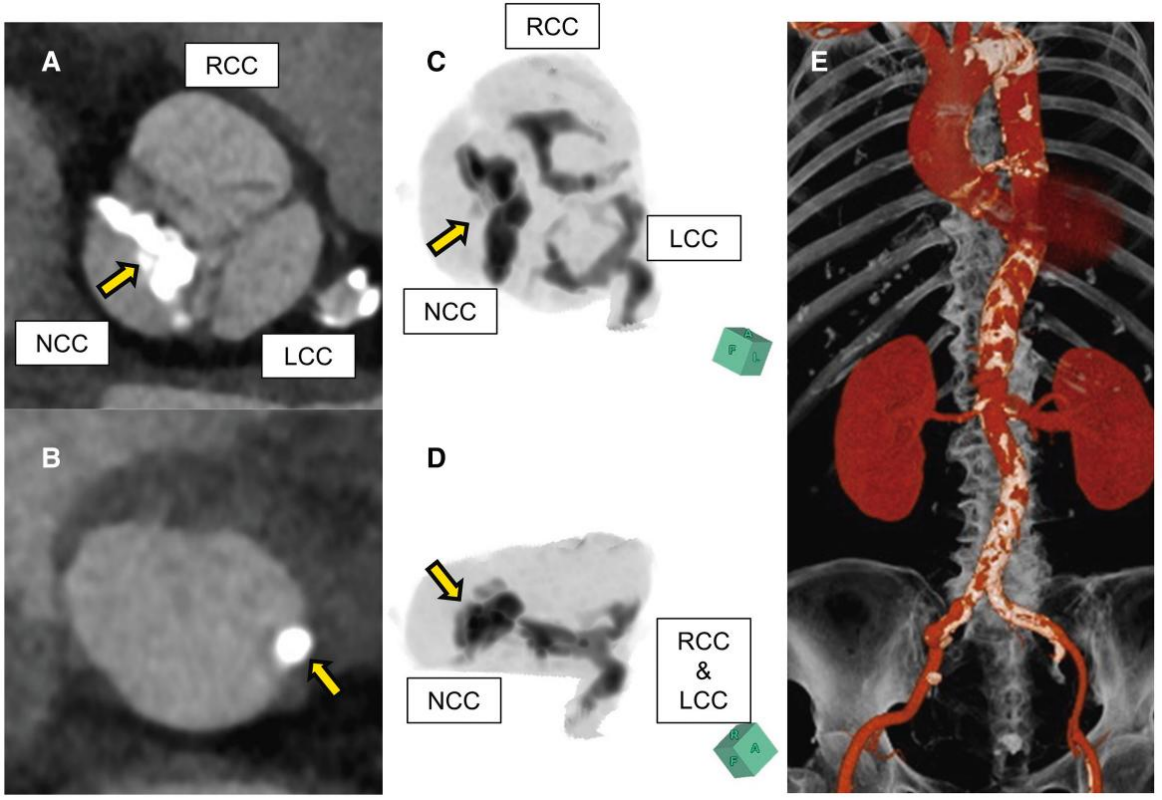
A pre-operative multislice computed tomography scan showed the following: Arrows indicate extensive calcification of the leaflet tips of the non-coronary cusps (*A*, *C*, and *D*). Arrows indicate continuous calcification of the left ventricular outflow tract (*B*). Computed tomography angiography revealed advanced calcification of the artery (*E*). NCC, non-coronary cusp; RCC, right coronary cusp; LCC, left coronary cusp.
